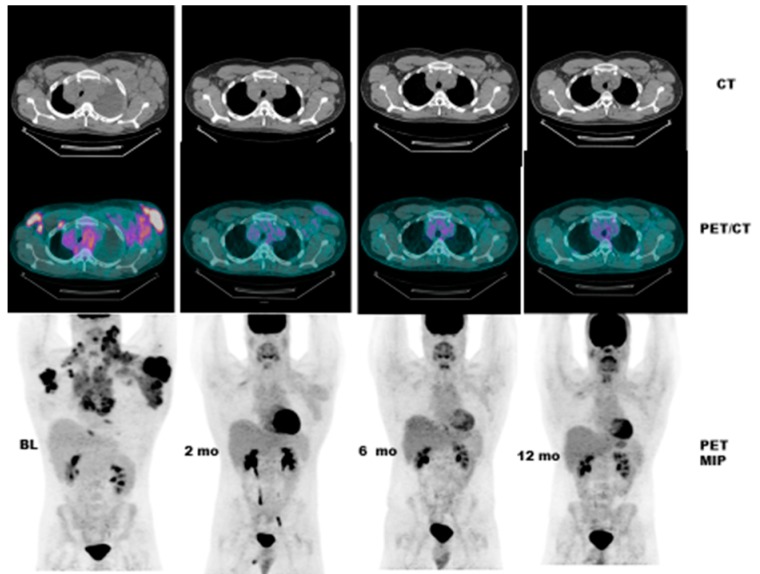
Pre and Post FDG PET CT in a 22 y/o male with Hodgkin lymphoma (nodular sclerosis type). He had complete metabolic response after 2 cycles of chemotherapy (ABVD) but only partial anatomic response. He completed 6 cycles of therapy with complete metabolic response but residual anatomic abnormalities. He had consolidation radiation therapy, about 1 month after completing chemotherapy; about 2 months after completing radiation therapy, there was no change. It is not uncommon to have residual masses after therapy for lymphoma, especially Hodgkin lymphoma, which may complicate anatomic response assessment.
